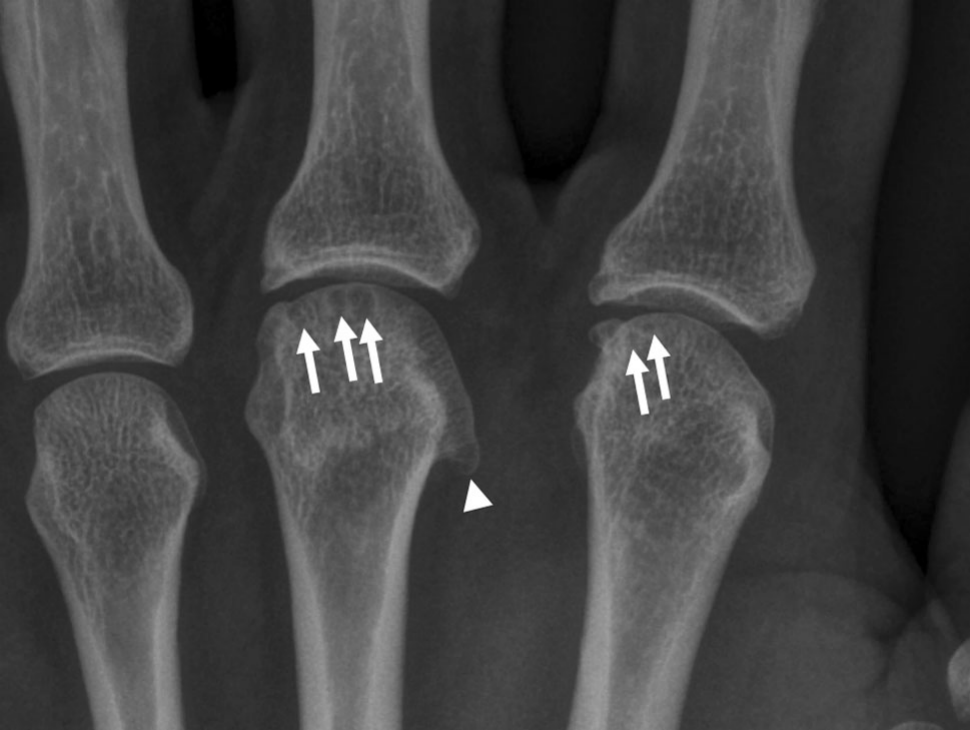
Radiography of the left hand with subchondral cysts and osteophytes in the 2nd and 3rd MCP joints (*arrows*), and overhanging and hook-like radial osteophyte in the 3rd metacarpal head (*arrow head*). The margins of these joints are irregular but the joint spaces were unremarkable. These findings were the same for both hands
